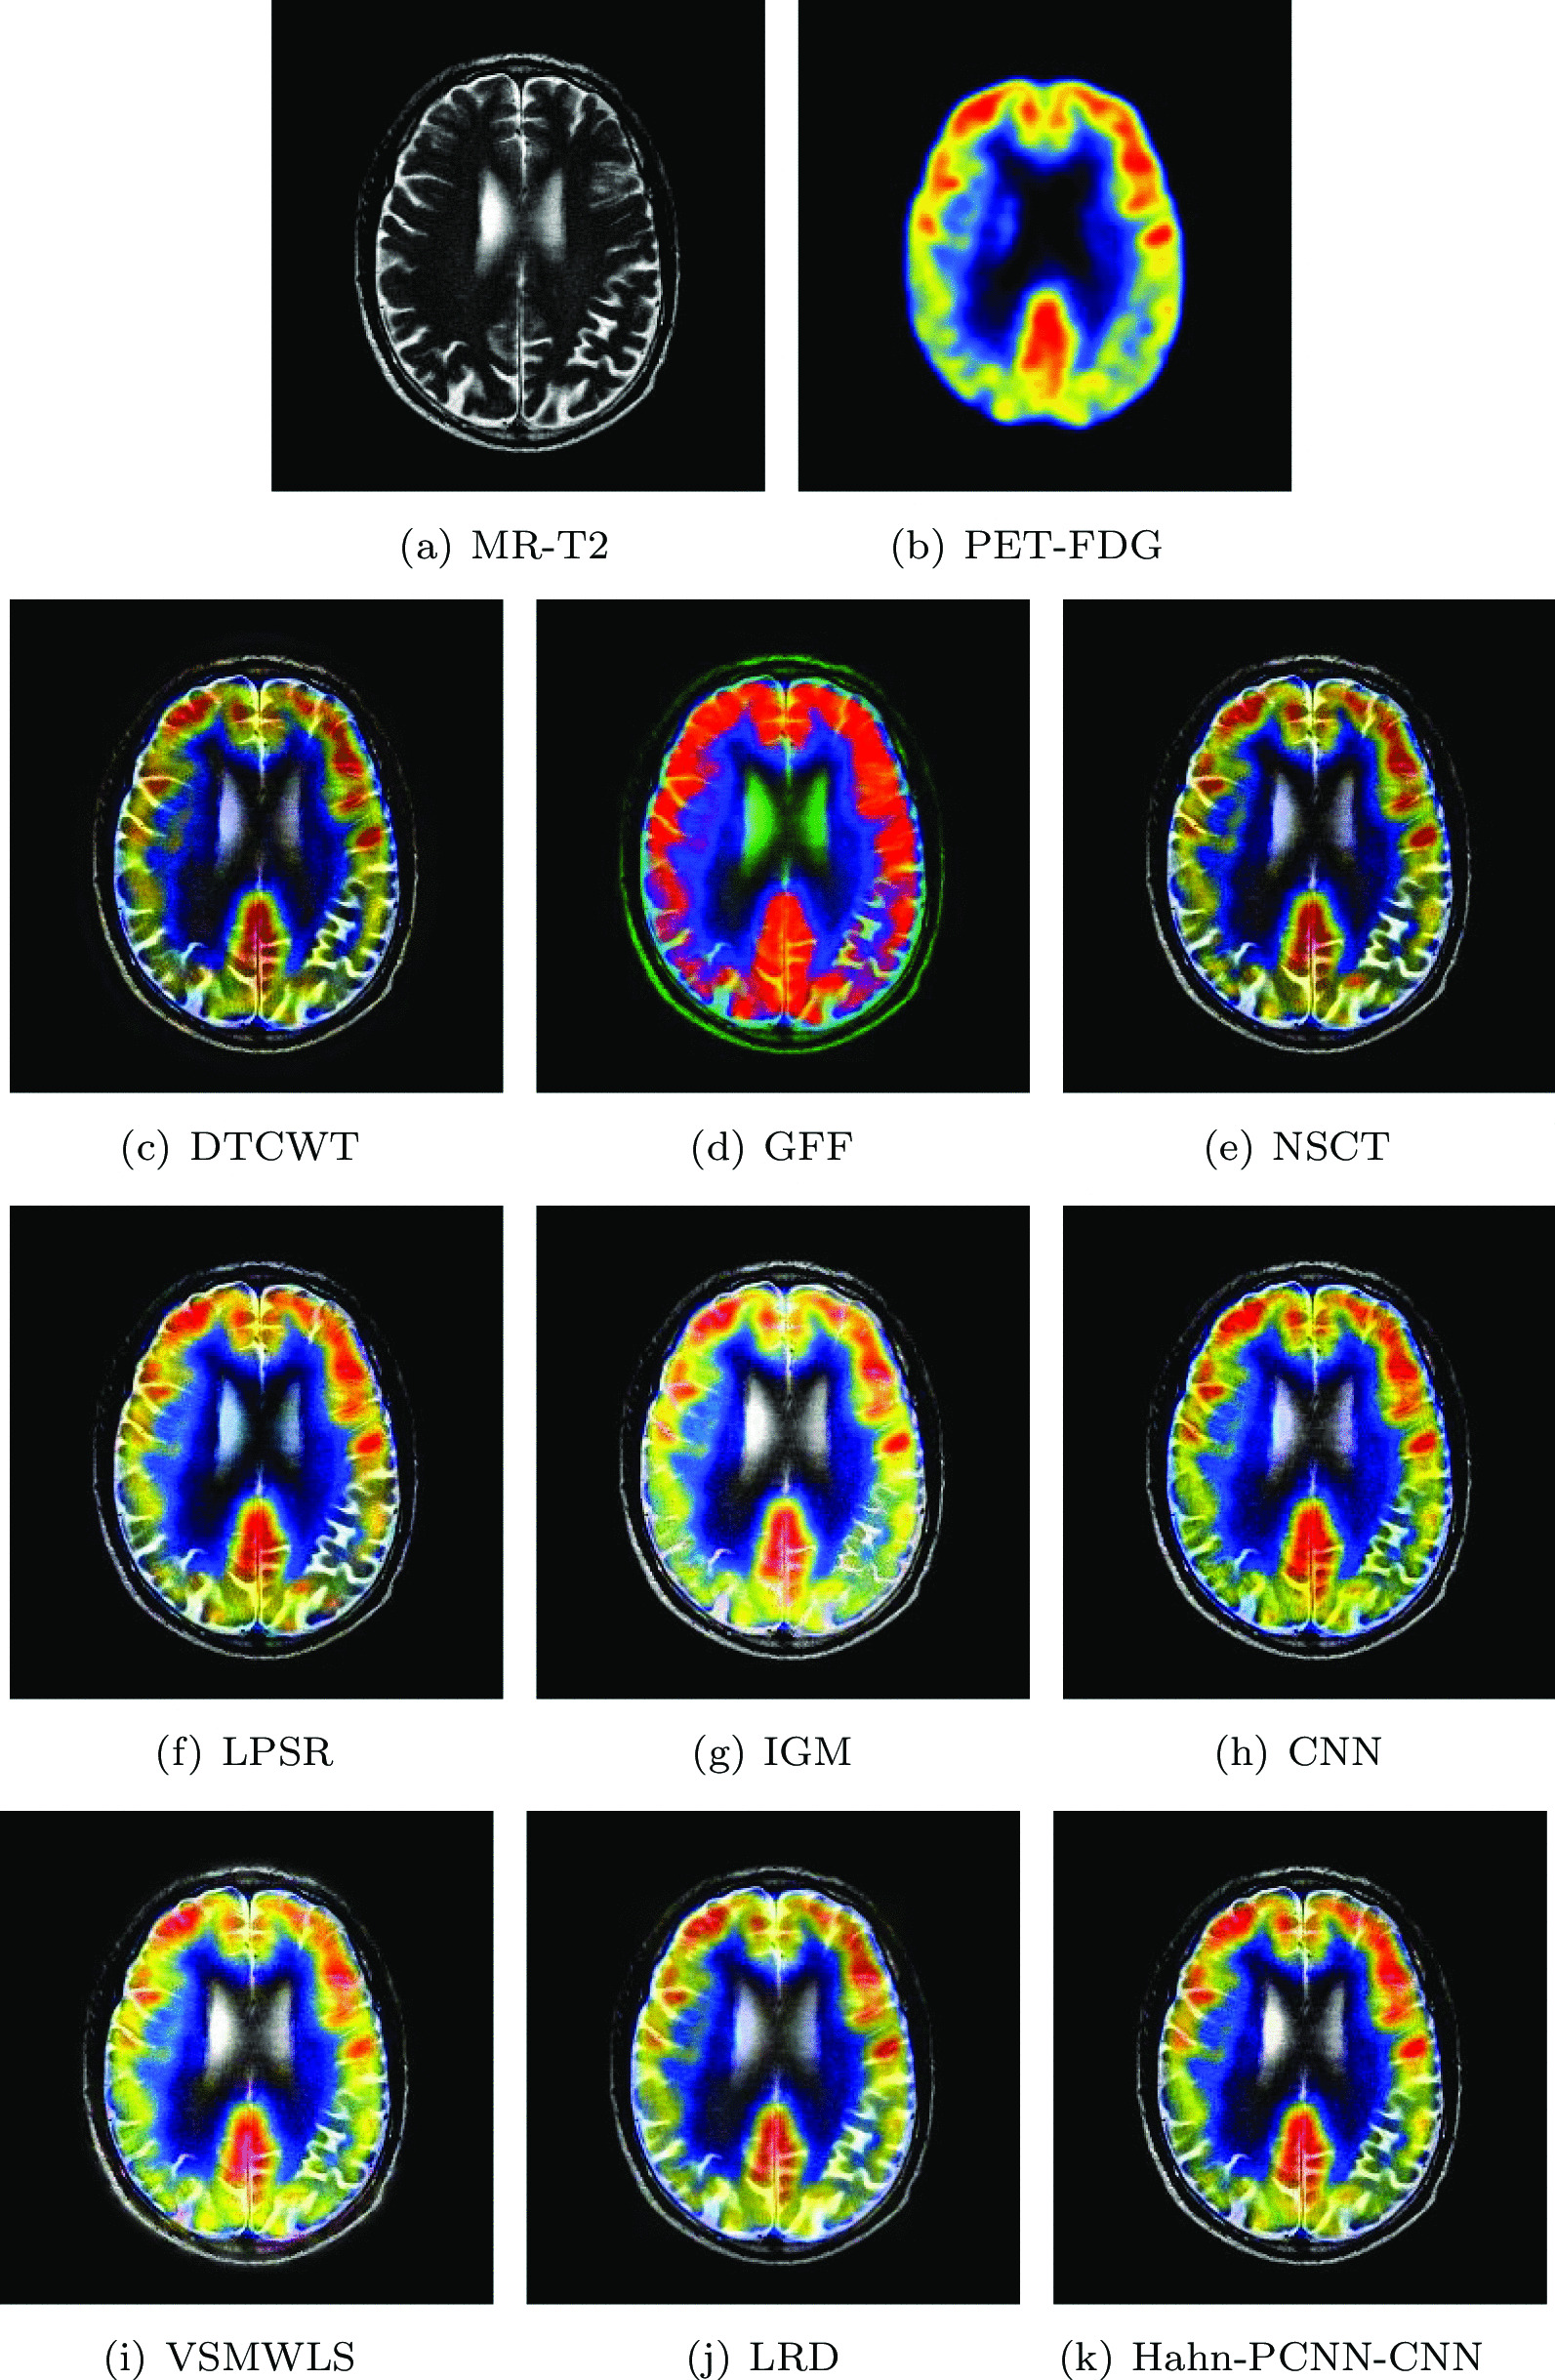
The second set of fused MRI-PET images from 9 methods on mild Alzheimer’s disease
